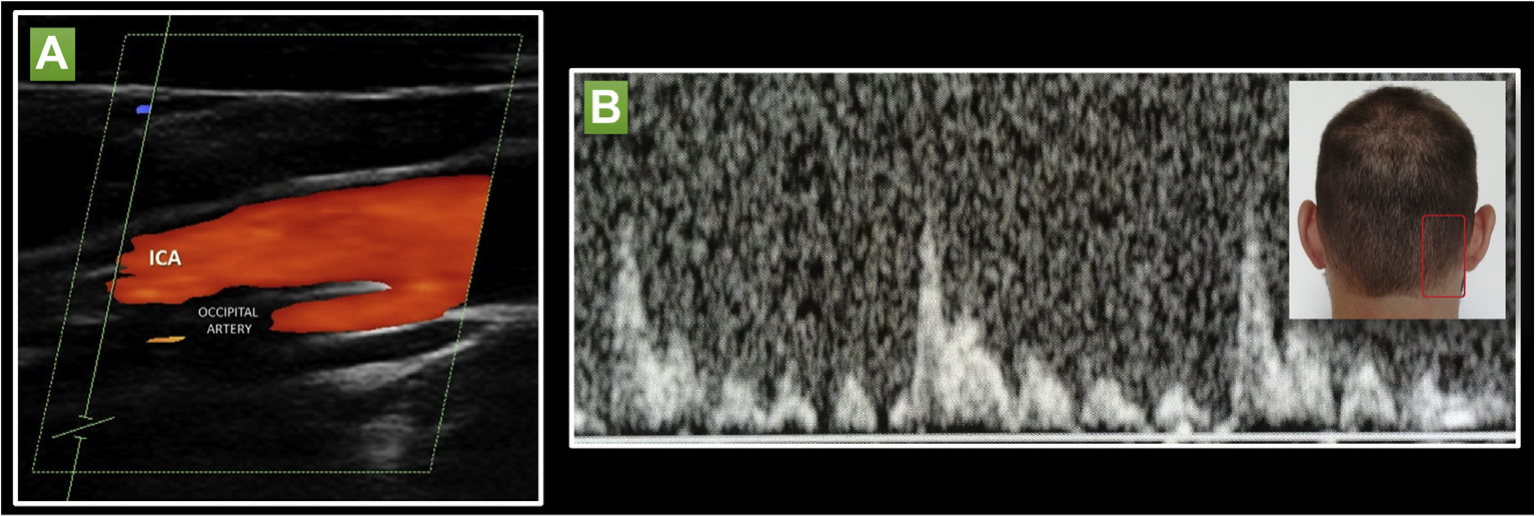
A, Carotid duplex ultrasound showing an internal right carotid artery double lumen. B, Occipital tap maneuver: during the assessment of the double lumen (occipital artery), tapping of the occipital artery produced a reflected oscillatory flow. A representative view of the tapping site in the occipital artery perfusion territory is shown (not a representation of the described patient).
